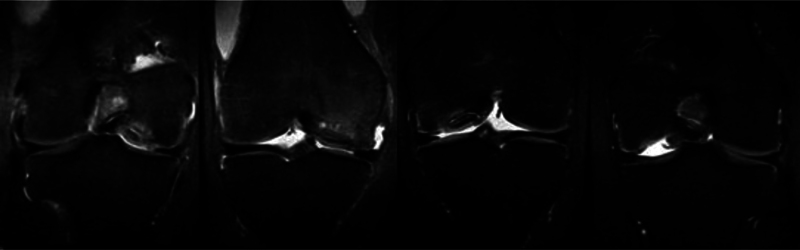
Coronal magnetic resonance imaging sequence showing the lesion in the medial femoral condyle of the right knee and left knee, respectively, resulting from osteochondritis dissecans.
